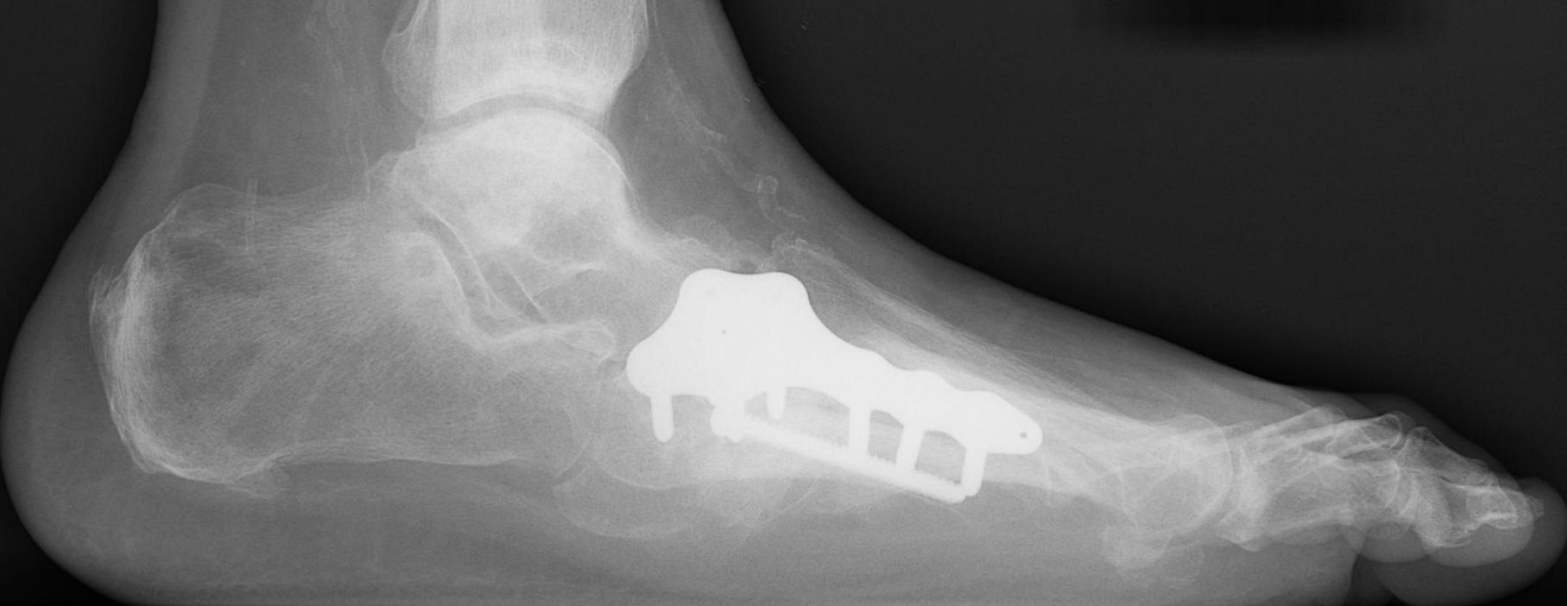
Lateral radiographic view showing anatomic alignment and consolidation across the arthrodesis sites.
